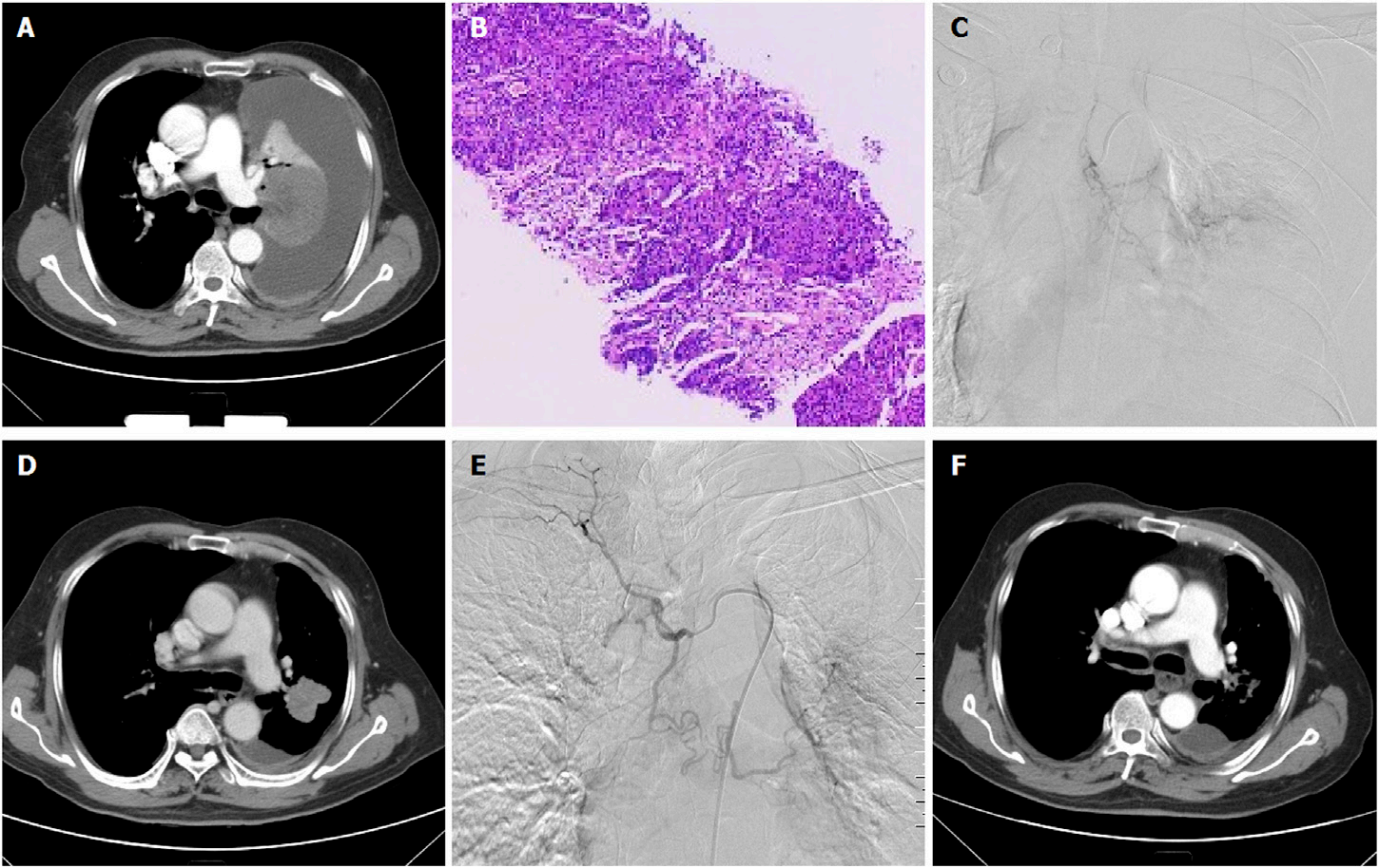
A 66-years male treated by CalliSpheres beads for advanced squamous cell carcinoma in left lung. (A) Computed tomography examination before procedure revealed malignant central tumor of left lung, with complete atelectasis and massive pleural effusion. (B) Pathological diagnosis of squamous cell carcinoma by biopsy of the lesion. (C) The left lung tumor was treated by CalliSpheres beads. (D) The left lung tumor shrunk and atelectasis disappeared 1 month after the first session of DEB-TACE. (E) The left tumor was treated again by drug-loaded beads 1 month later. (F) The tumor was almost disappeared 3 months after first DEB-TACE.
